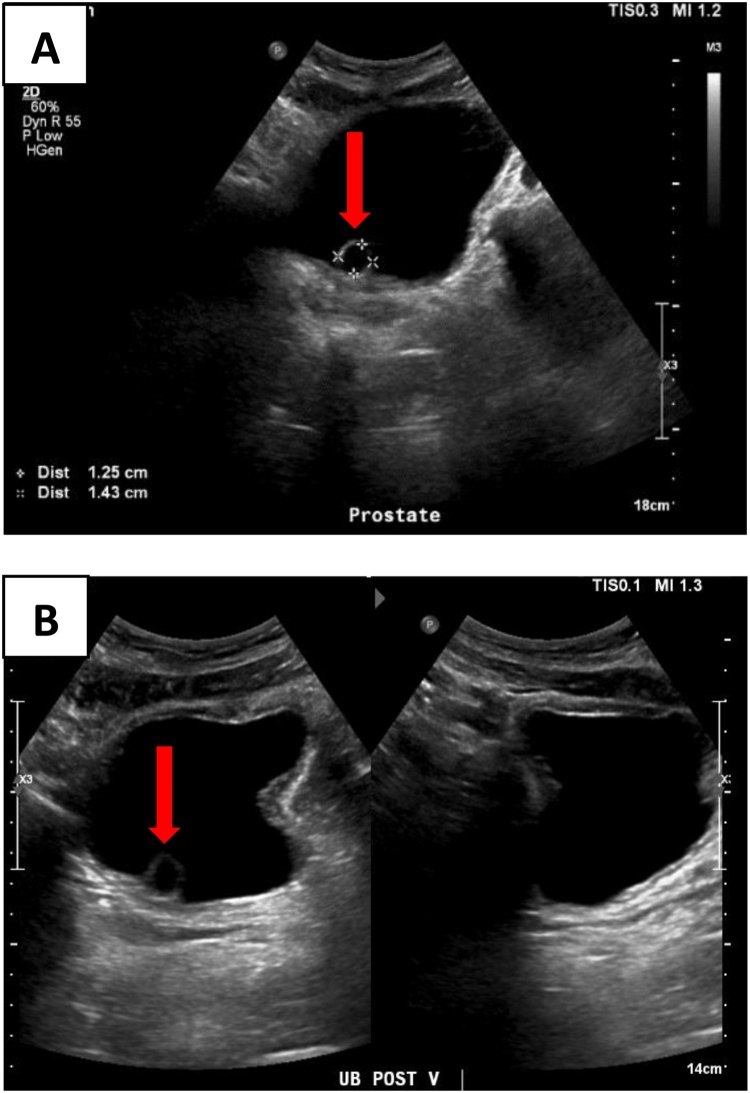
Pelvic ultrasound revealed a cyst measuring approximately 1.5*1.2 cm that was likely associated with the proximal part of the prostate gland and in close proximity to the proximal urethra (red arrow).
